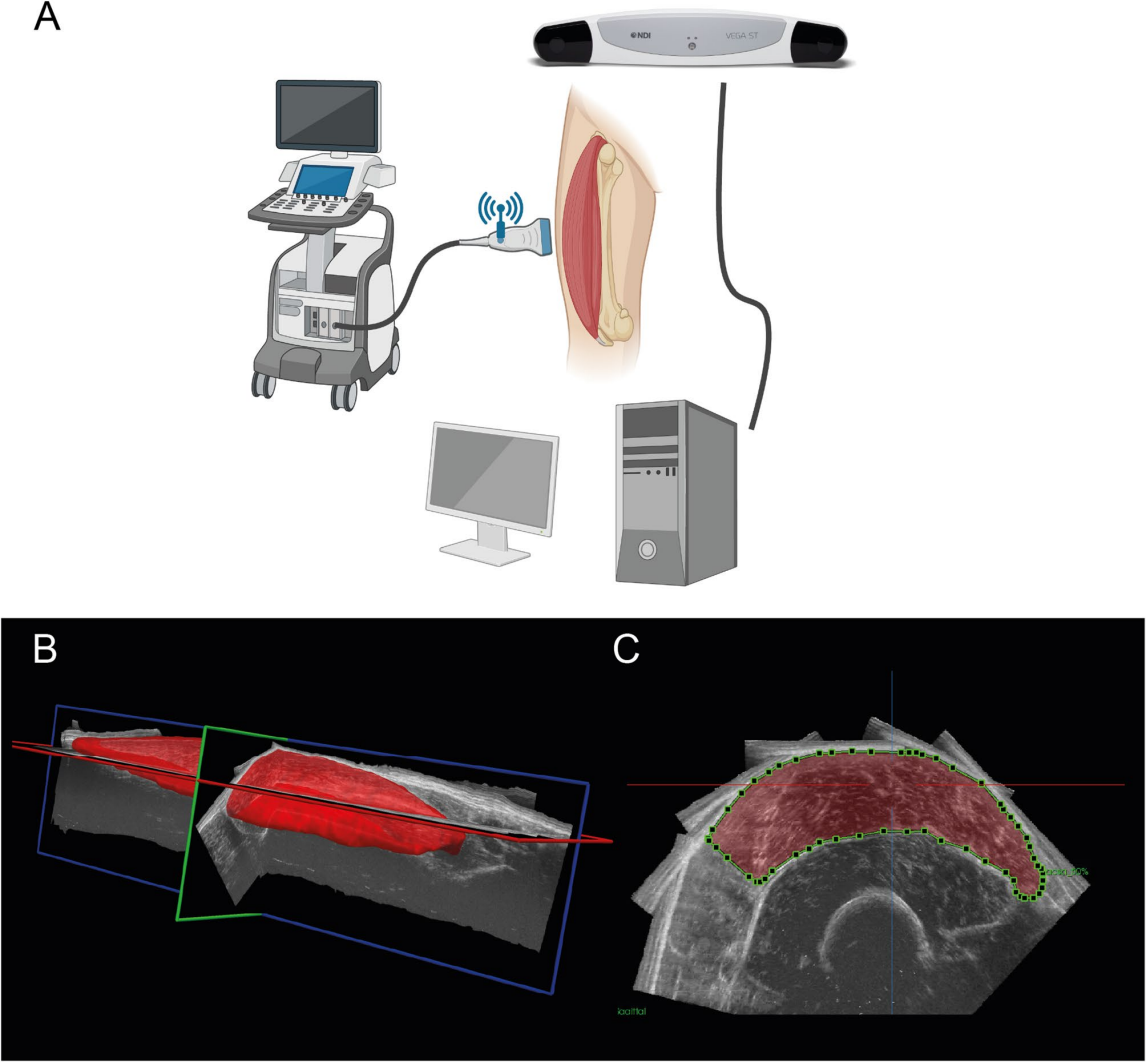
3D ultrasound methodology. (A) Ultrasound scans of the vastus lateralis were collected in longitudinal direction distally to proximally, covering the entire muscle belly. Location and orientation of the ultrasound probe were collected over time using a cluster marker set attached to the ultrasound probe, traced by a pose tracking device, enabling the construction of a 3D vastus lateralis muscle. (B) Representative example of a 3D ultrasound image reconstruction. (C) A segmented vastus lateralis anatomical cross-sectional area at 50% muscle length in the transversal plane using reconstructed images.
